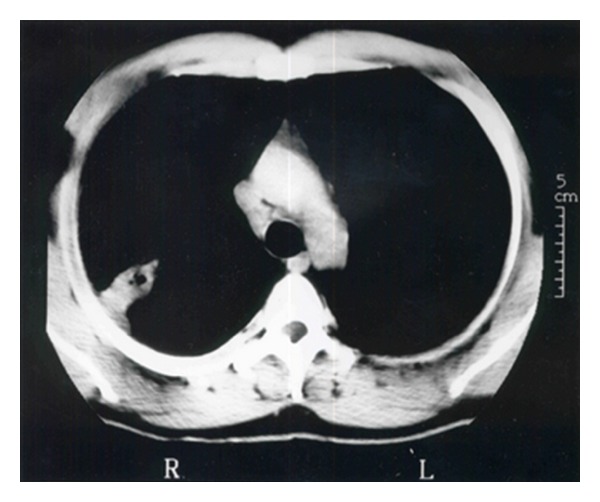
CT scan of the chest demonstrating the lesion in the posterior segment of the right upper lobe with central lucency.
